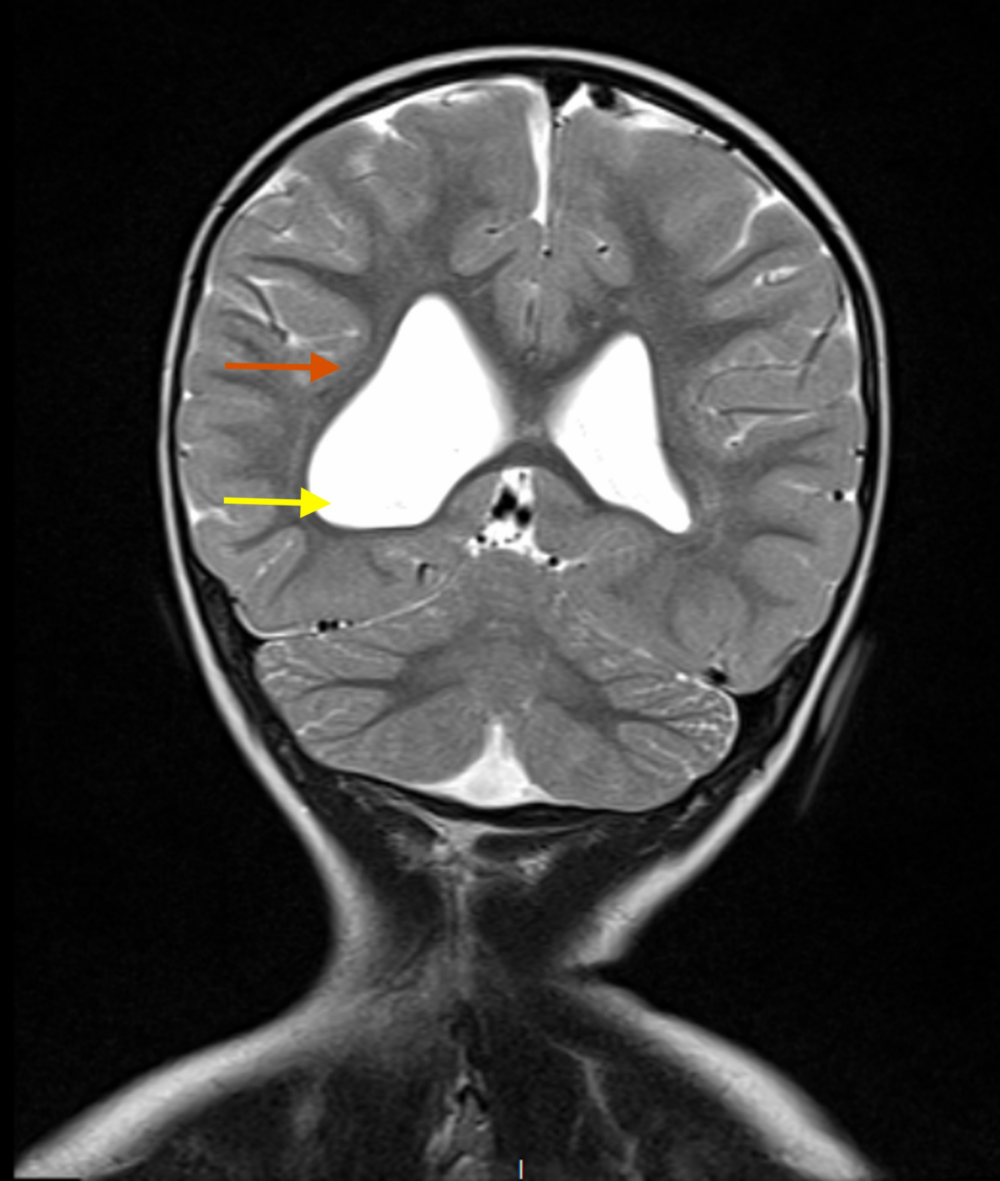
MRI of the head showing asymmetrical enlargement of the right lateral ventricle with predominant expansion of the posterior portion of the lateral ventricular body, the trigone and right occipital horn (yellow arrow), and white matter volume loss with marked thinning of white matter more pronounced in right side (red arrow).
